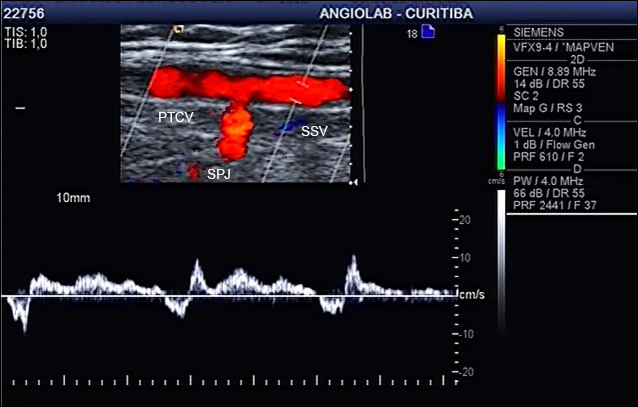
Ultrasound image of the small saphenous vein (same case as in Figure 6) with reflux, demonstrating that reflux in the Giacomini vein is transferred to the small saphenous vein. PTCV = Posterior thigh circumflex vein; SSV = small saphenous vein; SPJ = saphenopopliteal junction.
